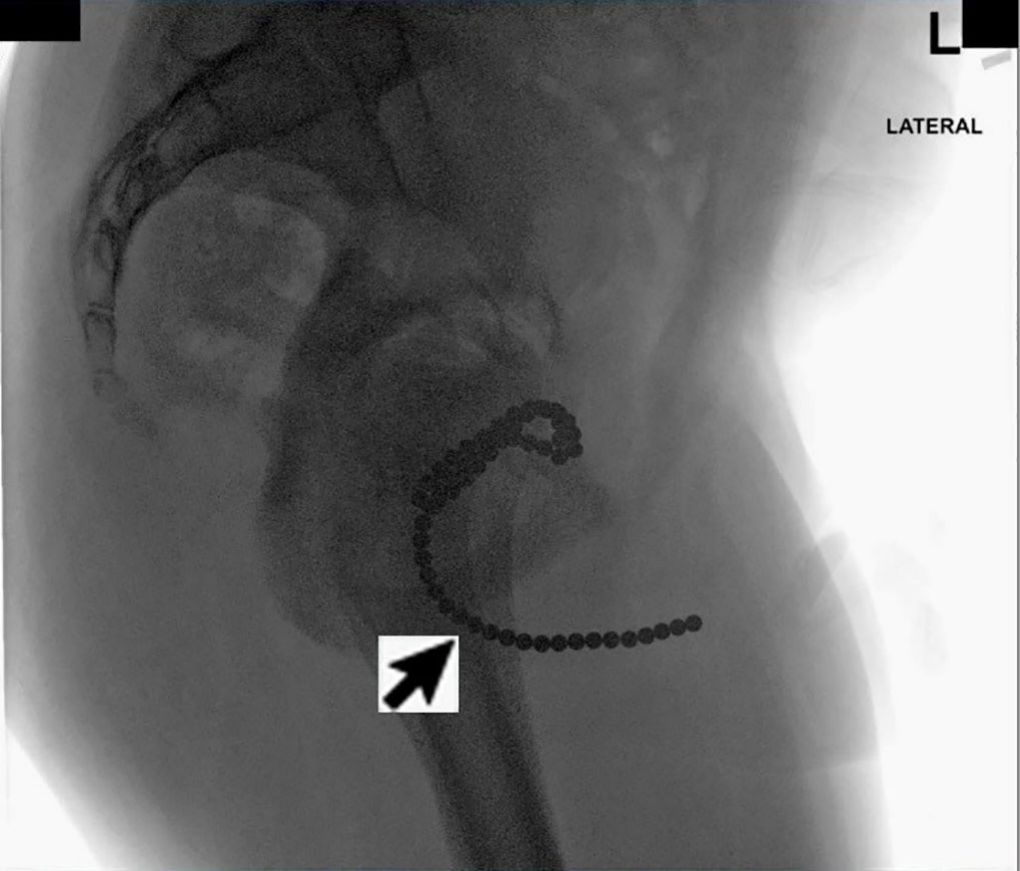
A lateral plain film radiograph of the pelvis demonstrating magnetic ball bearings in bladder and urethra.
